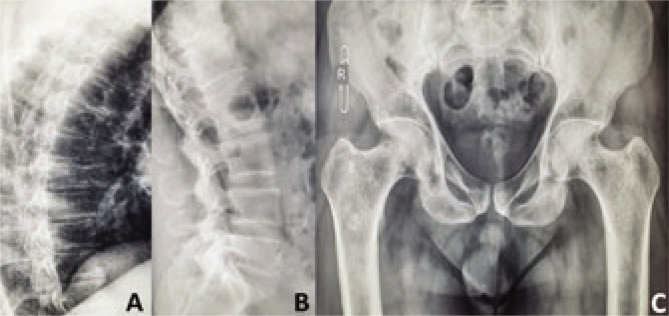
Plain radiographs of thoracic (A), lumbar (B) spine and pelvis-hips (C), showing diffuse osteopenia.
